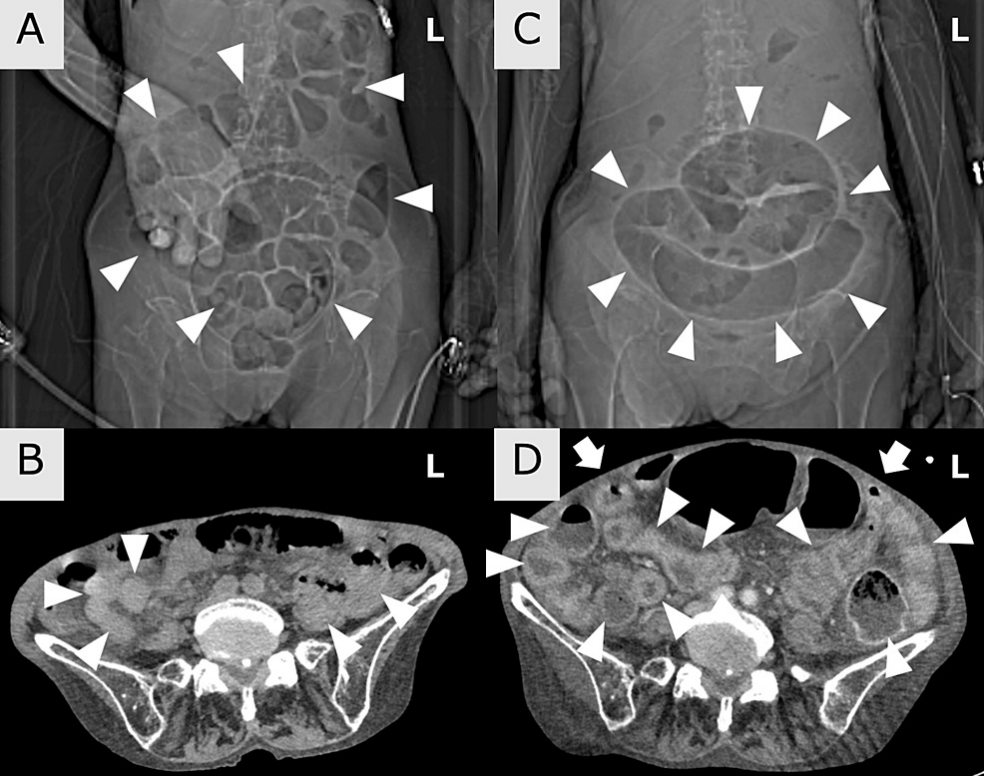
Imaging findings of this case A: On admission (day 1), a scout view of body computed tomography (CT) showed intestinal gas retention and a slightly distended bowel loop (arrowheads); B: Non-contrast-enhanced body CT showed mild intestinal edema (arrowheads); C: On day 5 after symptom deterioration, a scout view of the body CT showed an obvious distended bowel loop like coffee-bean sign (arrowheads); D: Contrast-enhanced body CT showed a distended abdomen (arrows) and intestinal edema (arrowheads).
